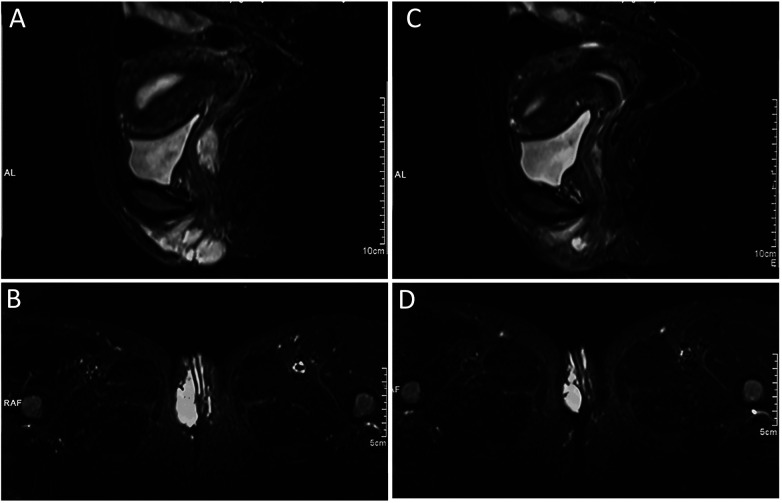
Representative preprocedural and postprocedural imaging findings in a patient with pelvic venous disorder and concomitant vulvar/external genital varicosities. (A,B) Preprocedural magnetic resonance imaging. (C,D) Postprocedural magnetic resonance imaging demonstrating interval improvement after treatment.
